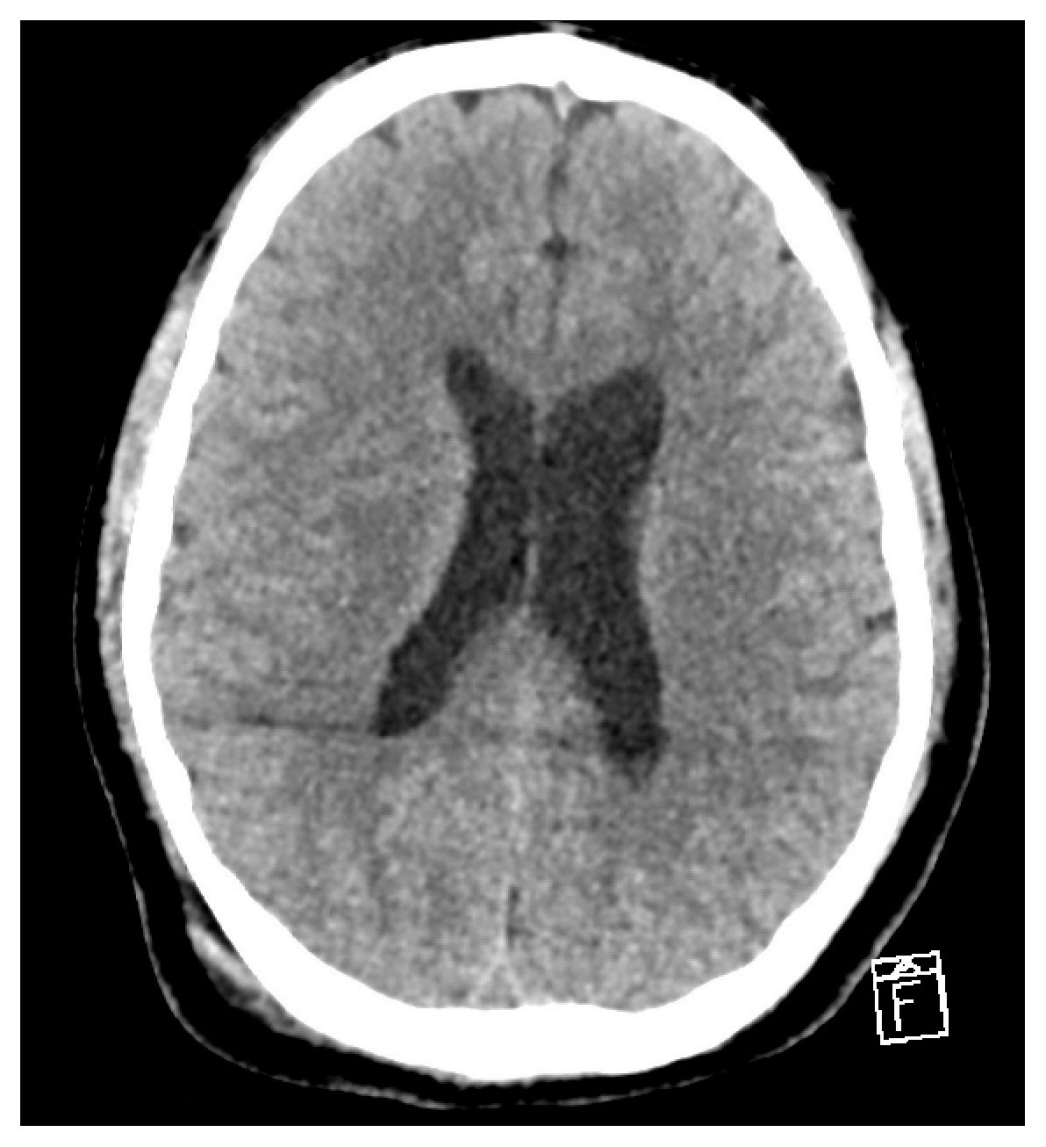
Axial non-contrast brain CT showing normal attenuation of the cerebral parenchyma with reserved gray-white matter differentiation. No mass effect or midline shift is noted.
